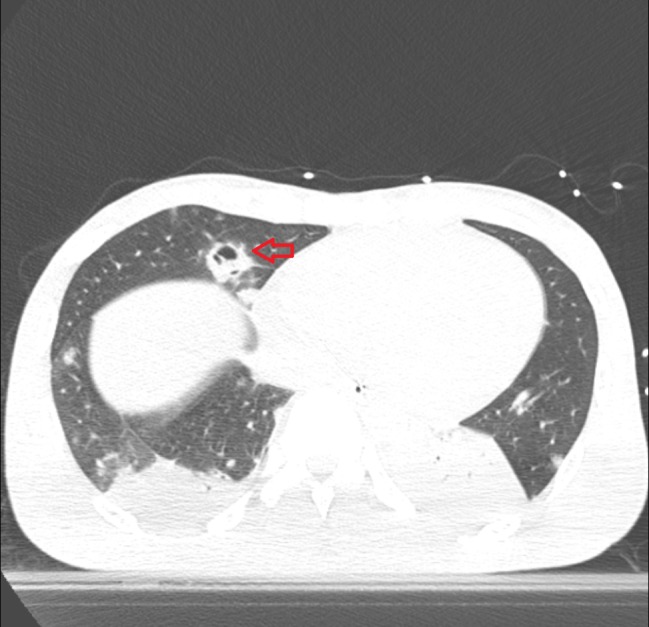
Computed tomography (CT) of the chest without contrast showing multifocal cavitary lesions seen throughout the lungs with largest cavitary lesion (read arrow) seen in the right middle lobe
